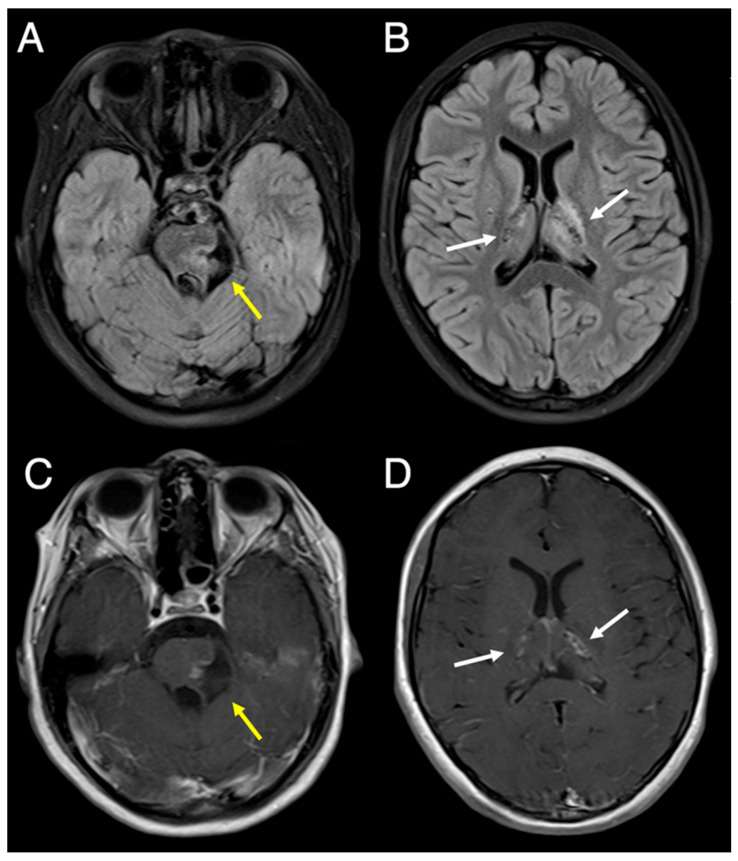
Axial 3T MRI slices one year after surgery at the level of the pons and basal ganglia, respectively. (A,B) Fat-saturated FLAIR images; (C,D) contrast-enhanced T1-TSE images show an appropriate degree of decompression of the brainstem cyst (yellow arrows) and stability of the basal ganglia lesions (white arrows).
